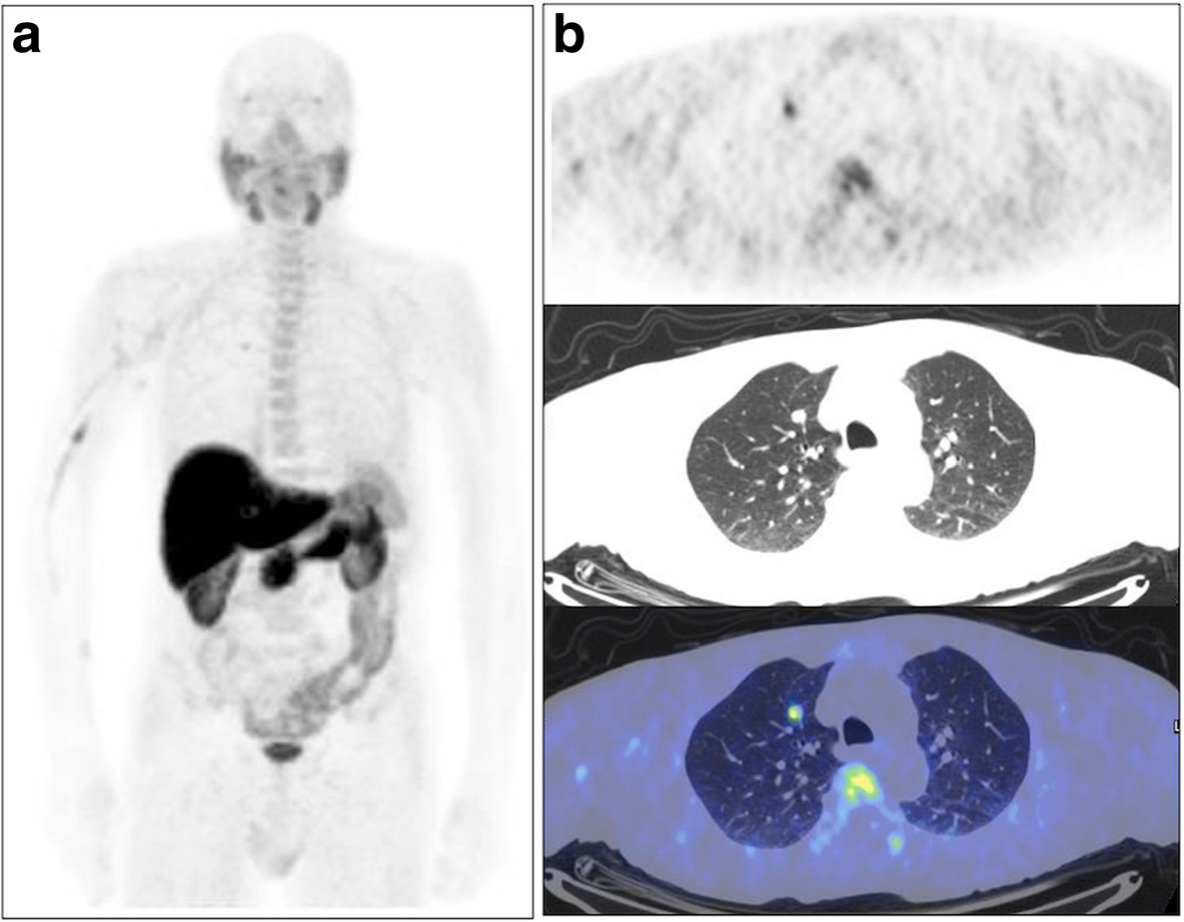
Case#2, 71-yr old man presenting raising PSA values after radical surgery for prostate cancer. The patient was also surgically treated for lung cancer. MIP (a) and axial (b) [^18^F]fluorocholine PET/CT images show an area of focal radiopharmaceutical uptake in the right lung, subsequently proven to be non small cell lung cancer
